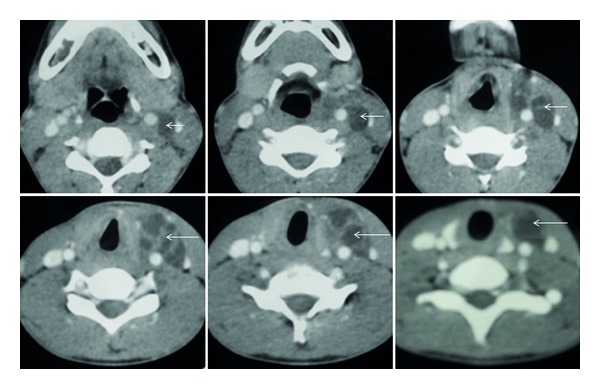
CT scan of the neck showed a multiloculated fluid density lesion with enhancing septae in the left parapharyngeal space, extending from the level of mandible up to C7 vertebral level.
